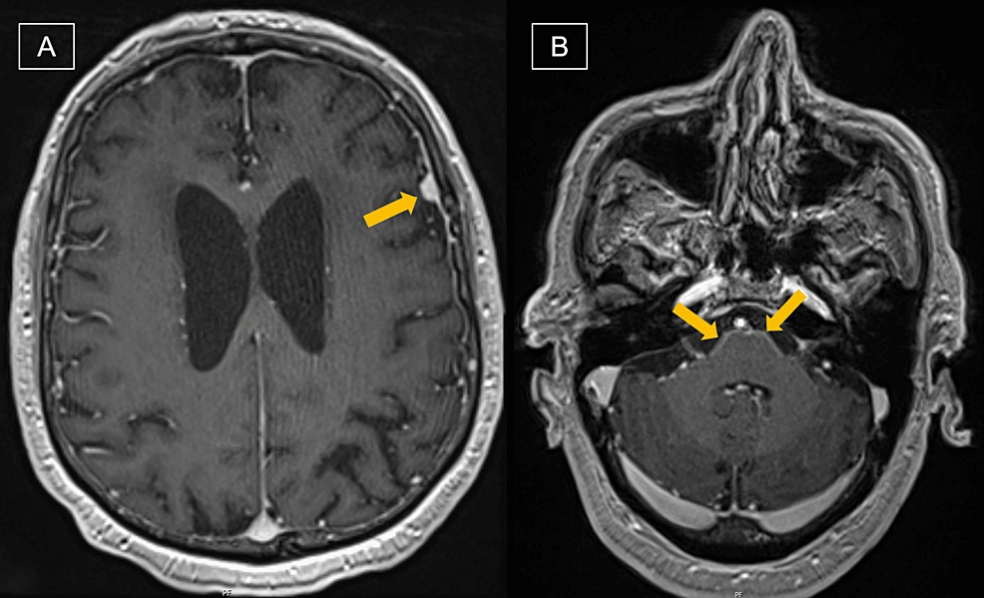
MRI brain with contrast (A) Bifrontal/bitemporal pachymeningeal enhancement with nodularity with a broad-based 7 mm nodule (arrow) and (B) leptomeningeal enhancement prepontine cistern (arrows)
